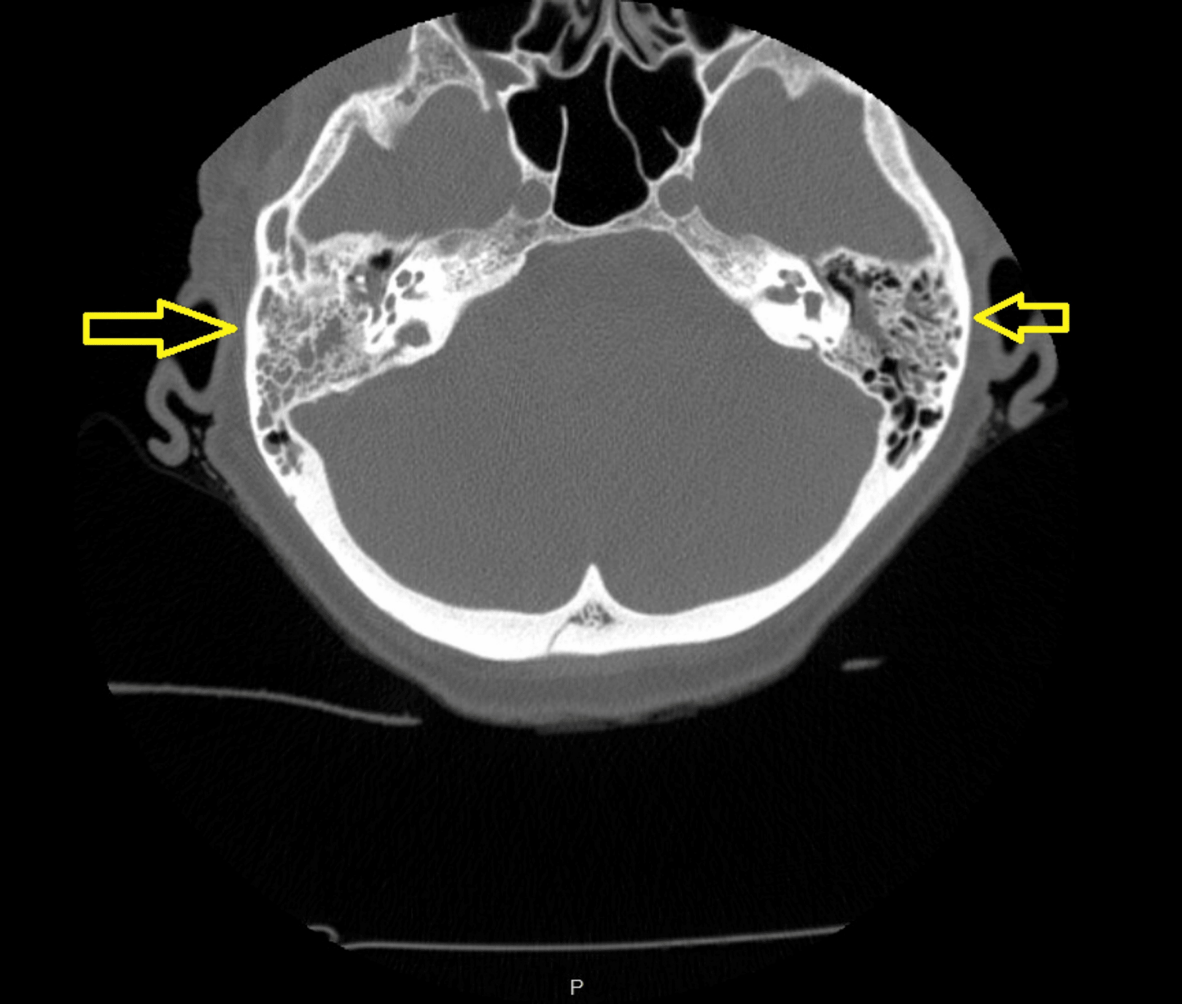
Findings from the patient’s computed tomography (CT) of the cervical spine indicating otomastoiditis, with a right-sided predominance as indicated by the larger arrow.
